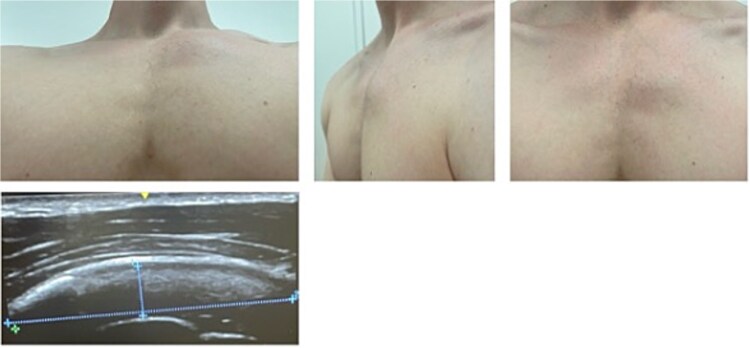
The young patient presenting in the chest wall outpatient clinic with severe pain and swelling of the third parasternal cartilage rib proportion. The ultrasound (bottom image) shows intact muscle tissue of the major pectoralis muscle but swelling just on top of the cartilage tissue.
